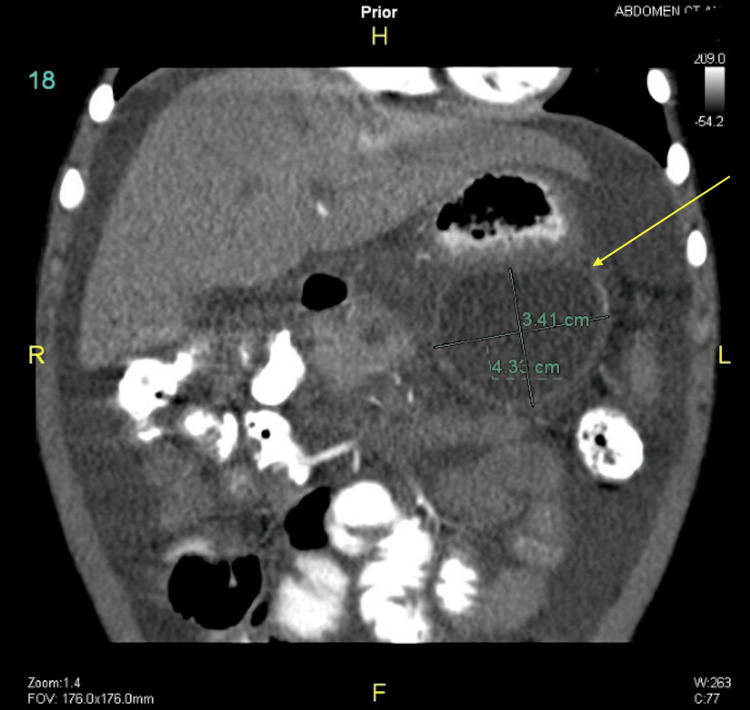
CT scan showing pseudocyst in the body and tail of the pancreas (arrow) CT: computed tomography
